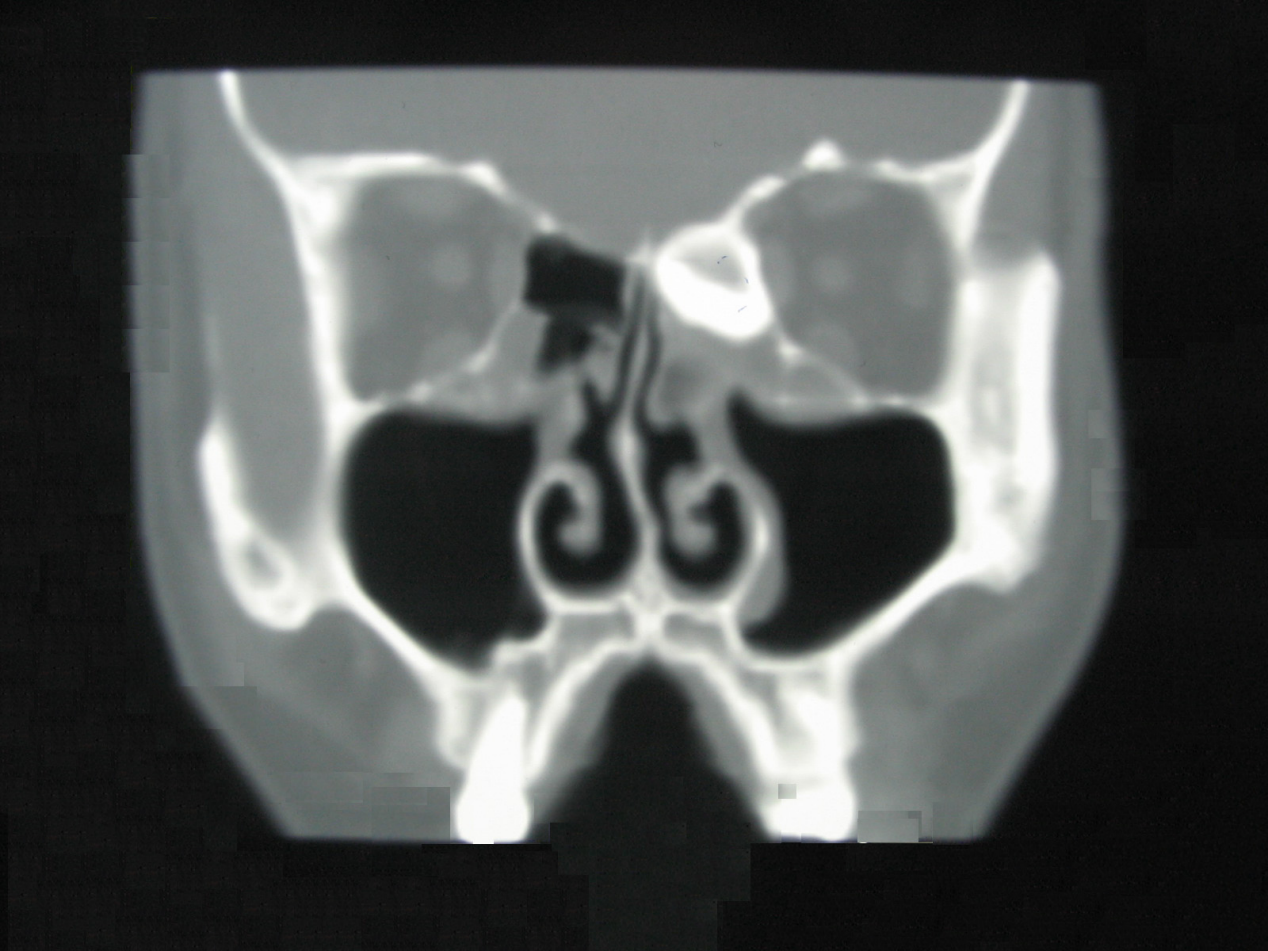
Ethmoid osteoma. CT revealed a high-density bony shadow in his left ethmoid sinus.
